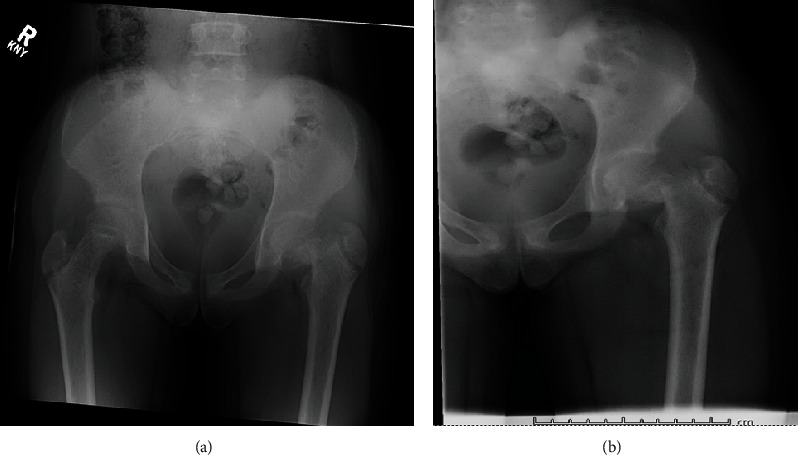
Initial X-rays demonstrating a Delbert type III left-sided femoral neck fracture.
